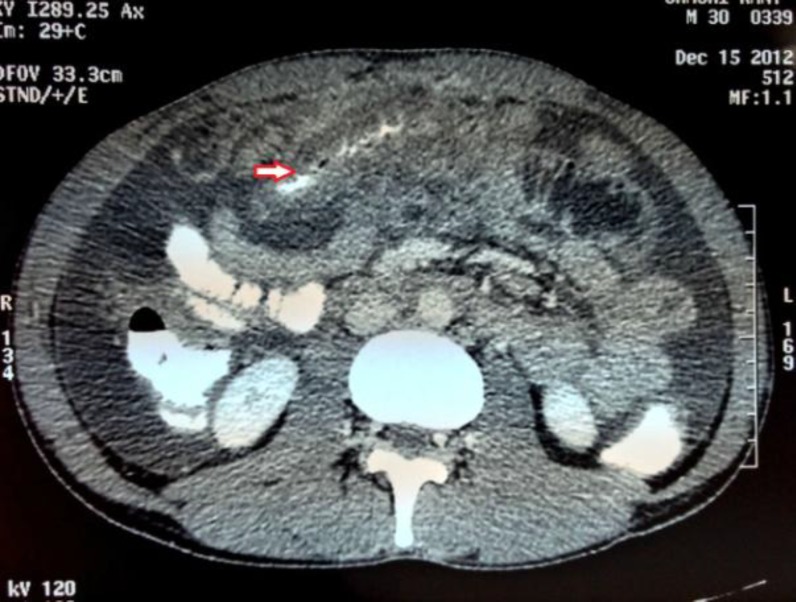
Contrast enhanced CT imaging of abdomen showing intestinal thickening of small gut (jujenum) and ascites
